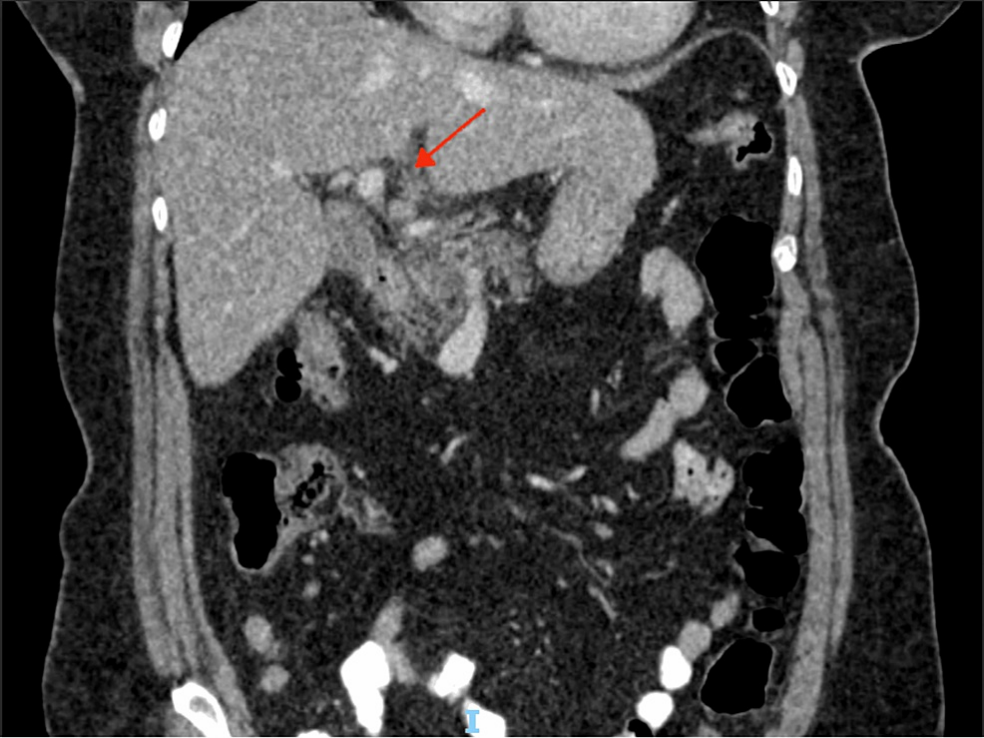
Coronal view of the CT scan of the abdomen and pelvis, showing fat stranding in the left subhepatic space centred around the falciform ligament.
